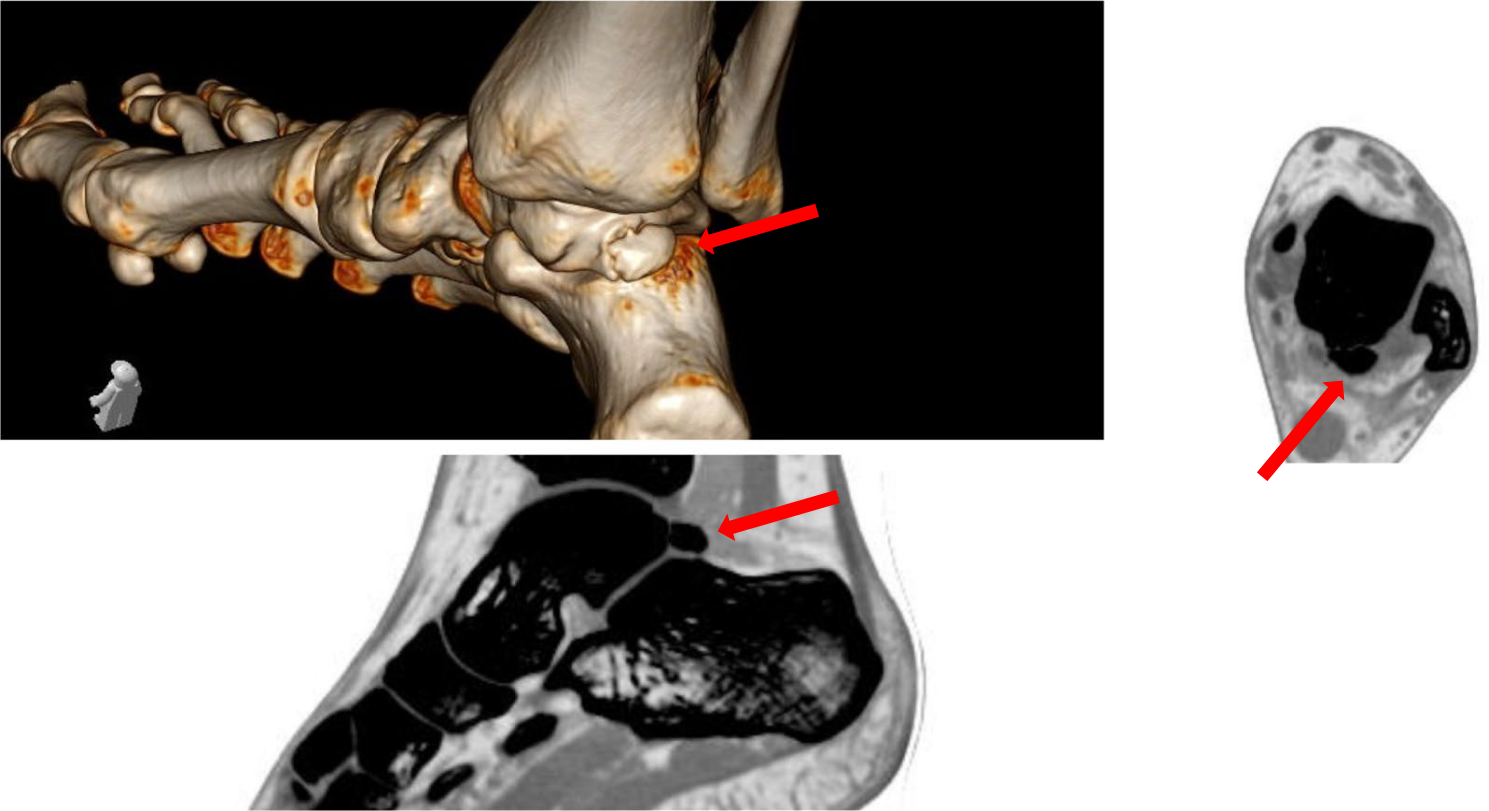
Preoperative volume rendering (VRT) CT and multiplanar reconstructions permitting better visualization of the fracture, the degree of displacement and comminution of the posterior process. Based on these factors surgical decision is made either for osteosynthesis or fragment excision. The red arrow points to the fracture site
